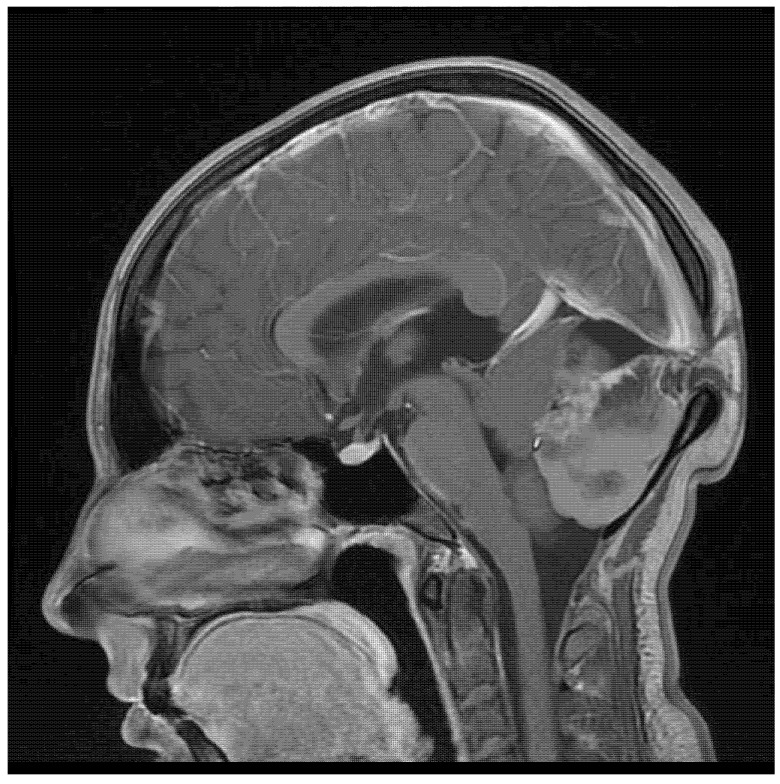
A sagittal contrast-enhanced T1-weighted image showing the tumor with bone loss, through which a tumor peduncle is passing, forming its extracranial part.
